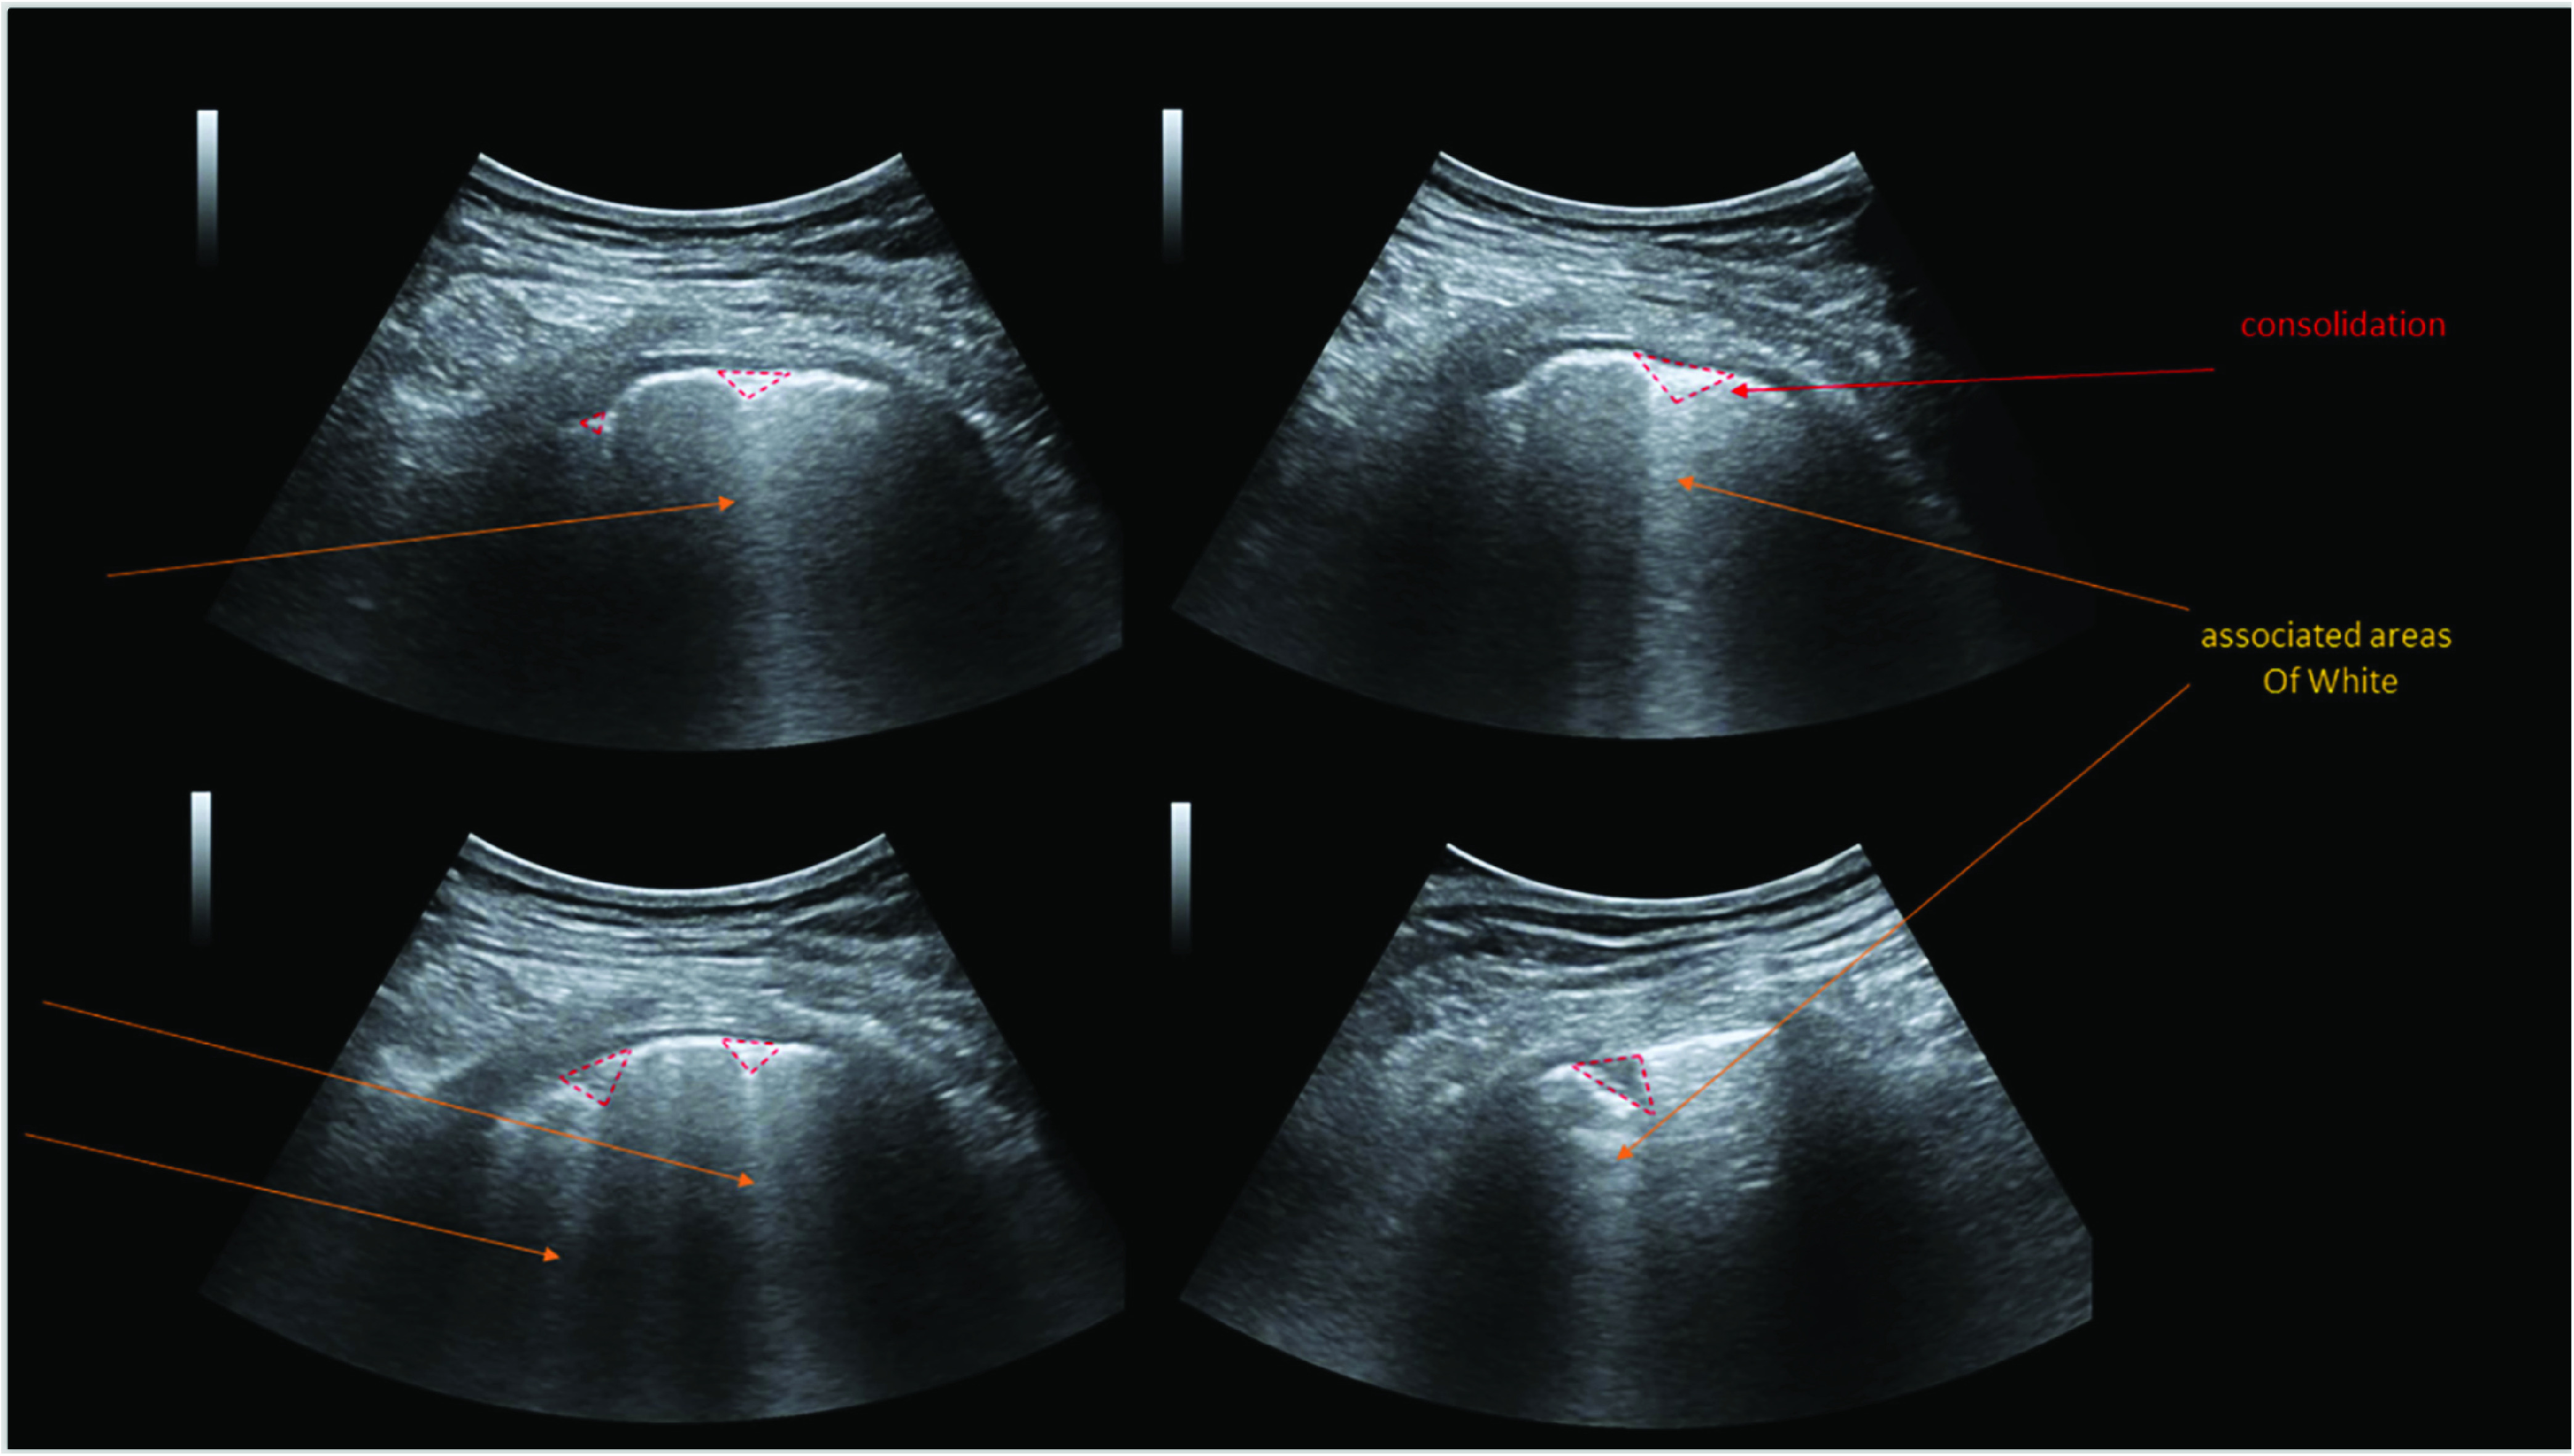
Detection of COVID-19 from ultrasound images: Ultrasound imagery is widely available and accessible throughout the world and therefore, can be a valuable tool for monitoring disease progression. Adapted from [55].
